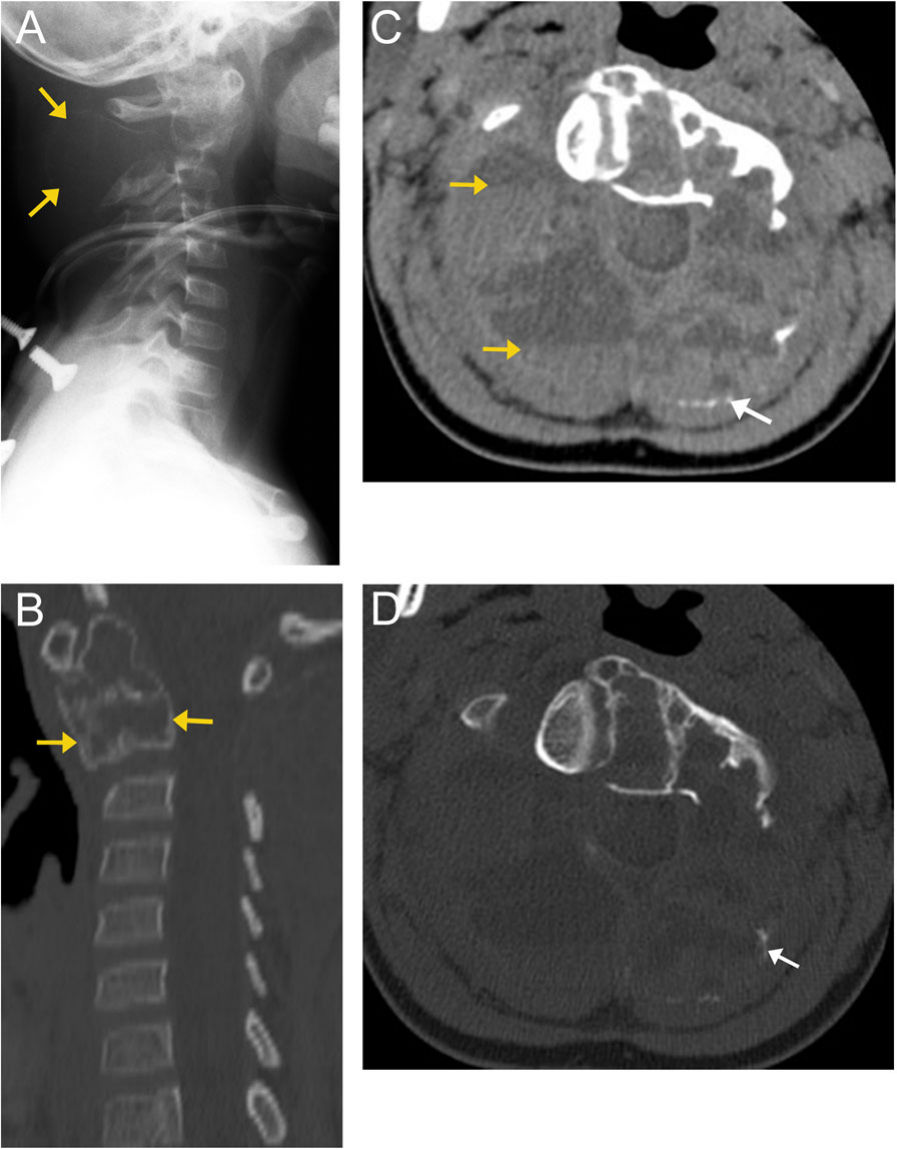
Aneurysmal bone cyst. Lateral radiograph (a) of the cervical spine demonstrates a large, osteolytic, expansile mass arising from the C2 vertebral body, resulting in significant bony remodeling. The mass is sharply defined and demonstrates thin sclerotic margins, also known as an “eggshell cortex” (orange arrows). Sagittal CT image of the cervical spine (b) confirms the lesion within the C2 vertebral body. Axial CT image in the soft tissue window (c) shows fluid-fluid levels (orange arrows), compatible with hemorrhage. Axial CT image in the bone window (d) shows near complete obliteration of the posterior arch with some areas of remnant thin sclerotic rim, "eggshell cortex" (white arrow)
